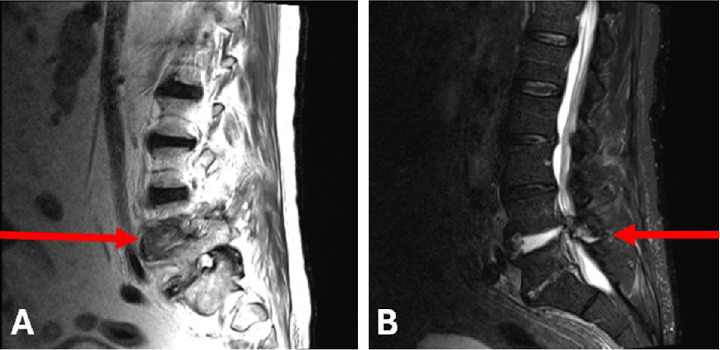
T1 and T2 STIR images of the MRI of the lower back show extensive discitis secondary to *Enterobacter cloacae* infection.
